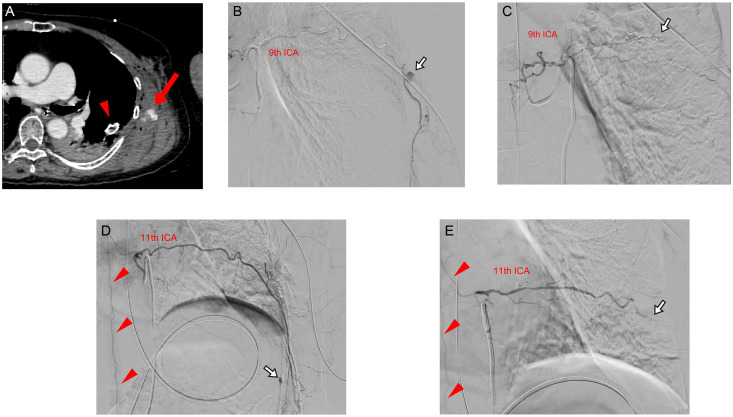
(A) Contrast-enhanced computed tomography (CT) after injury showed an extravasation of contrast media (arrow) from the chest wall. An arrowhead represents an aspiration tube inserted into the thoracic cavity for the left hemothorax. (B) Angiography revealed an extravasation (arrow) from the left 9th intercostal artery in the mid-portion at the rib fracture level. No Adamkiewicz artery was observed. (C) Angiography following embolization using gelatin sponge particles and metallic coils revealed complete occlusion of the artery (arrow). (D) Angiography revealed an extravasation (arrow) of contrast media from the left 11th intercostal artery in the mid-portion at the rib fracture level. The Adamkiewicz artery (arrowheads) originates from the proximal portion of the artery, but the vessel was not recognized at the time of the procedure. (E) Angiography after embolization with gelatin sponge particles and metallic coils showed complete occlusion of the artery (arrow), while the Adamkiewicz artery (arrowheads) remains patent.
